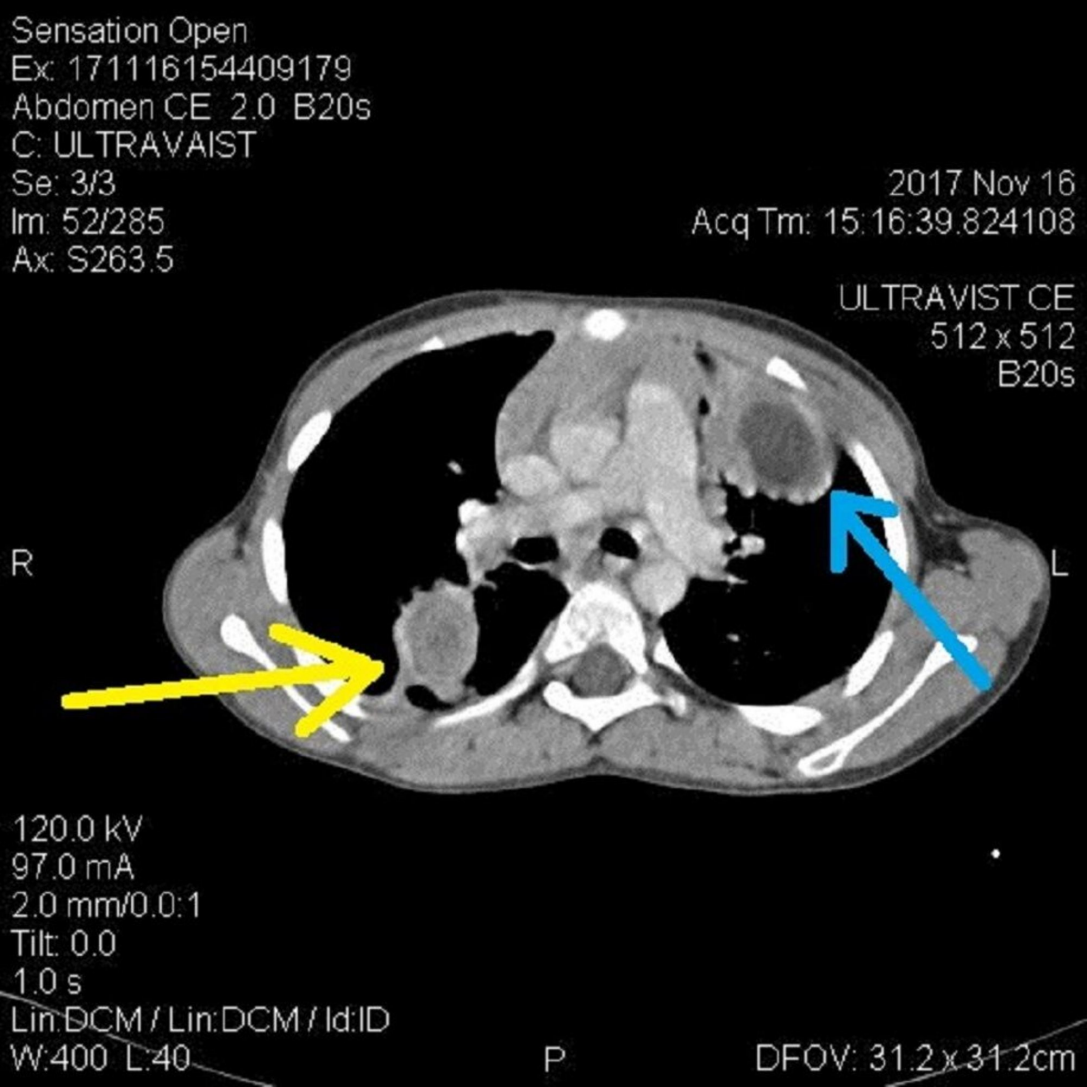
Computed tomography (CT) findings Cystic areas in left lower lung lobe (blue arrow) and apical segment of right lower lobe (yellow arrow).
